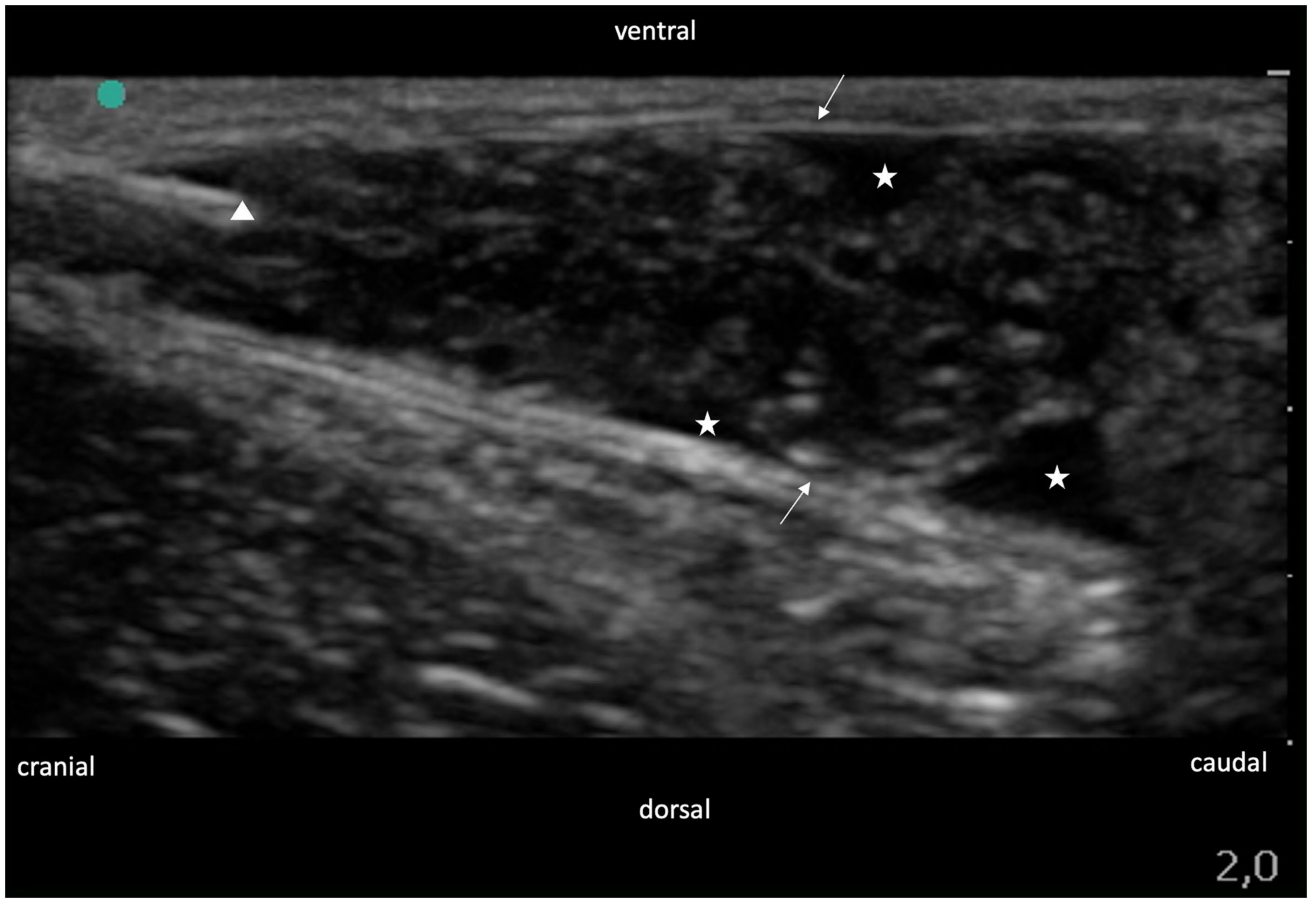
Ultrasonographic image for the visualisation of the injection site for the UGIF block. Arrowhead: tip of the hypodermic needle; stars: local anaesthetic that has already been injected; between arrows: spermatic cord.
